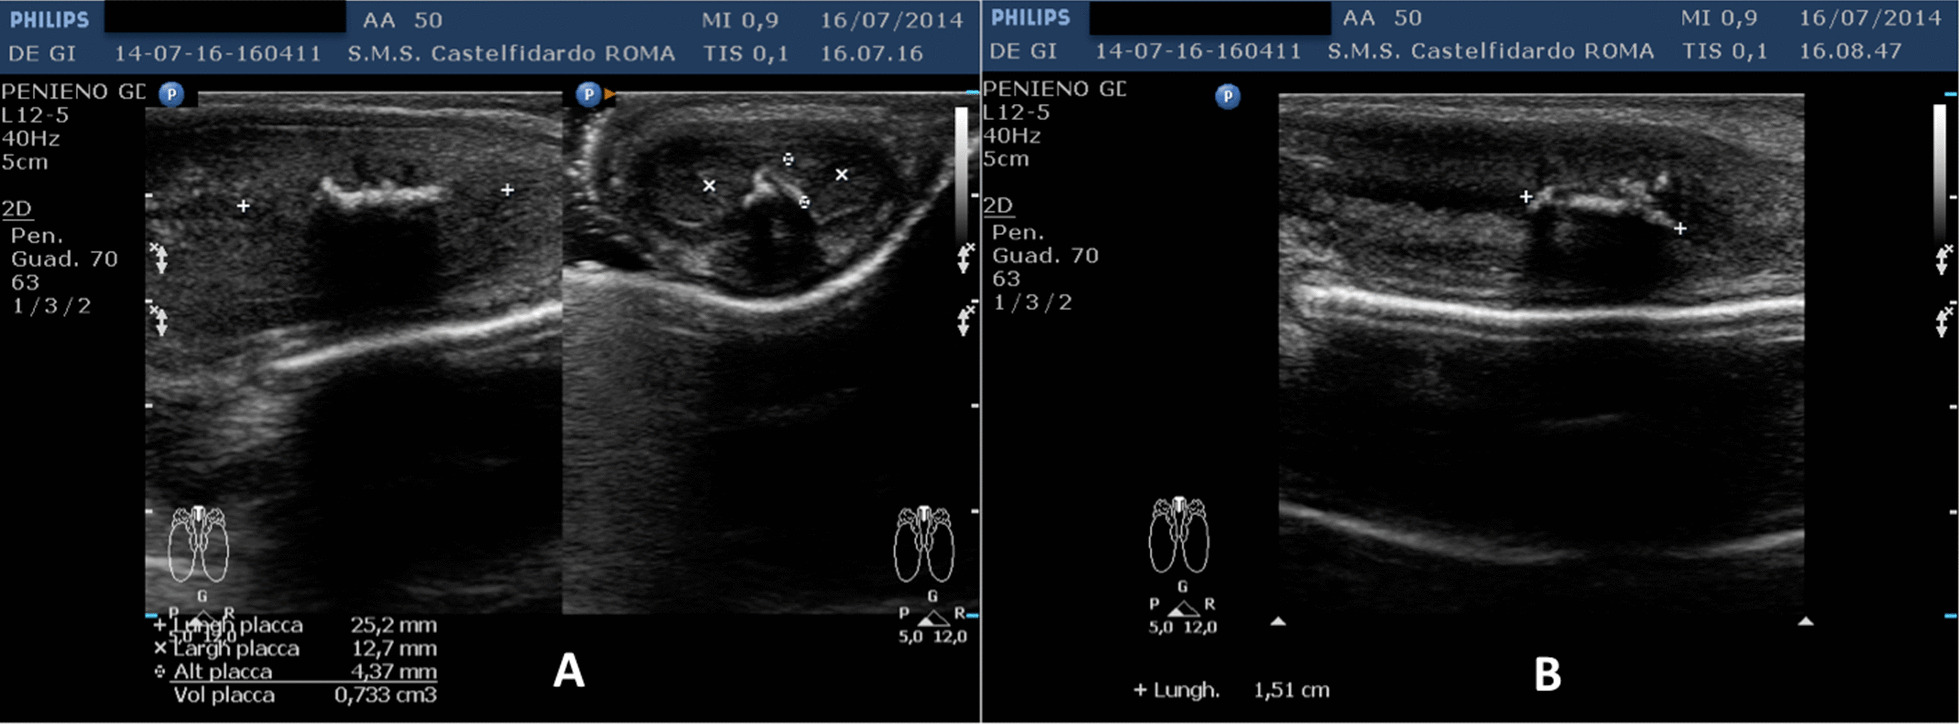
Penile ultrasound prior to treatment. A Plaque measurement in longitudinal and transverse scan. B Calcification measurement in longitudinal scan
